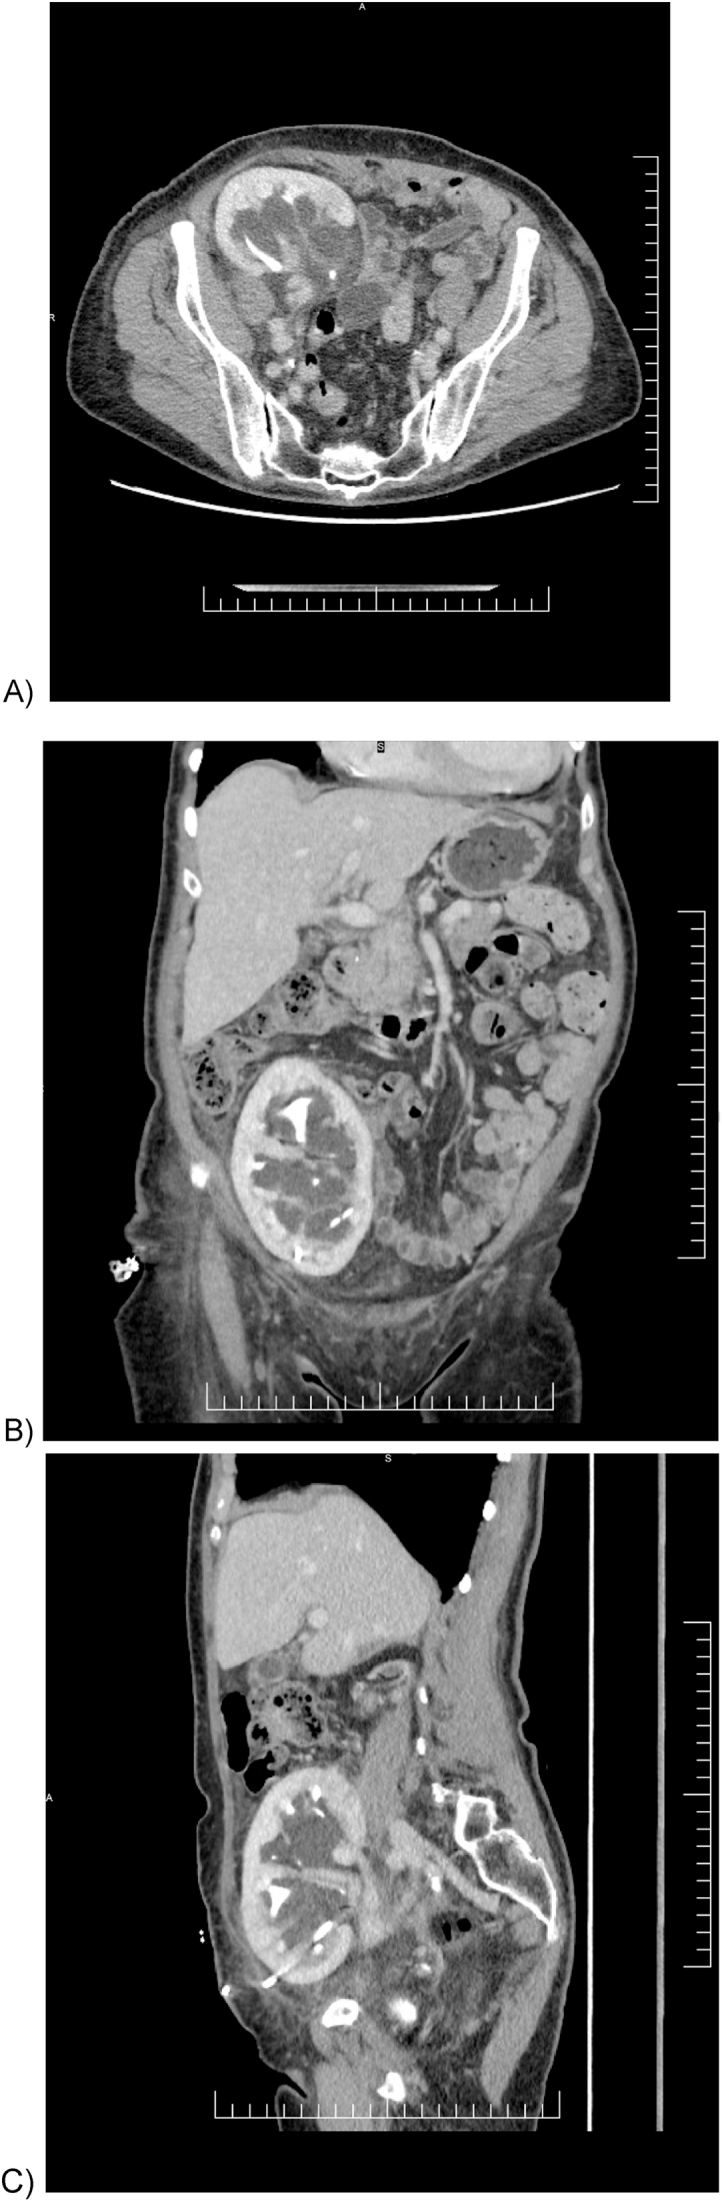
Contrast-enhanced computed tomography intravenous pyelogram (CT IVP) demonstrating the renal allograft with multiple parapelvic cysts, correctly placed nephrostomy tube, and persistence of graft “hydronephrosis”. (October 2024). A) axial, B) coronal, C) sagittal views.
